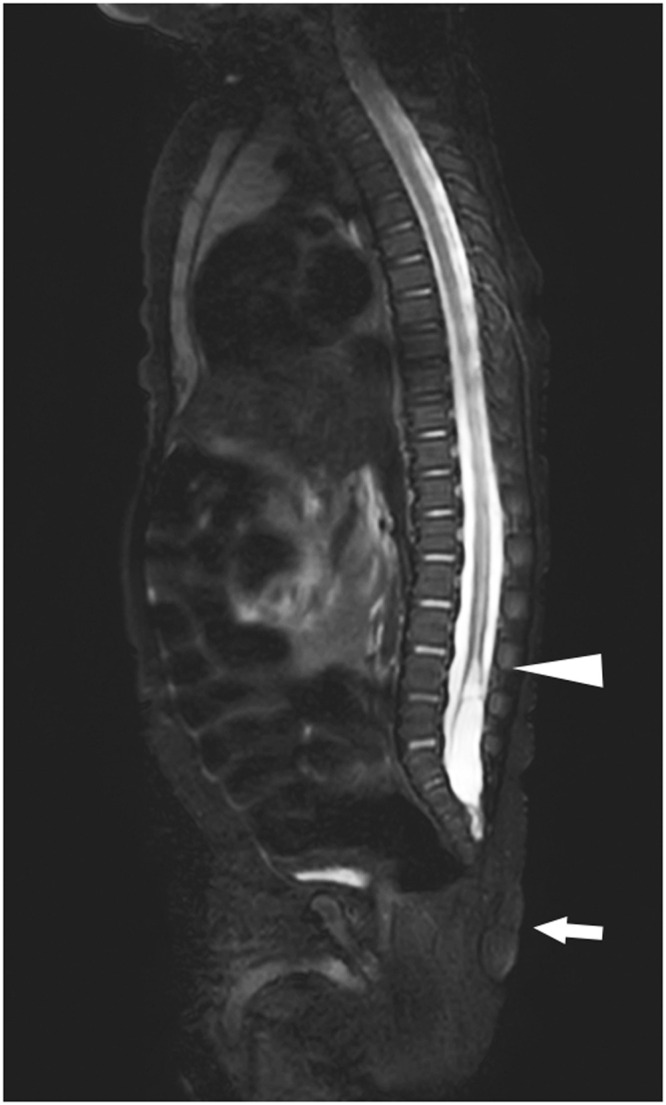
Spinal MRI findings (T2-weighted images with fat suppression). No continuity was observed between the human tail and the spinal canal or its internal structures. The arrow indicates the location of the tail. A low-lying conus medullaris is visible at the L3 level (arrowhead), with cystic expansion at the terminal portion of the spinal cord.
